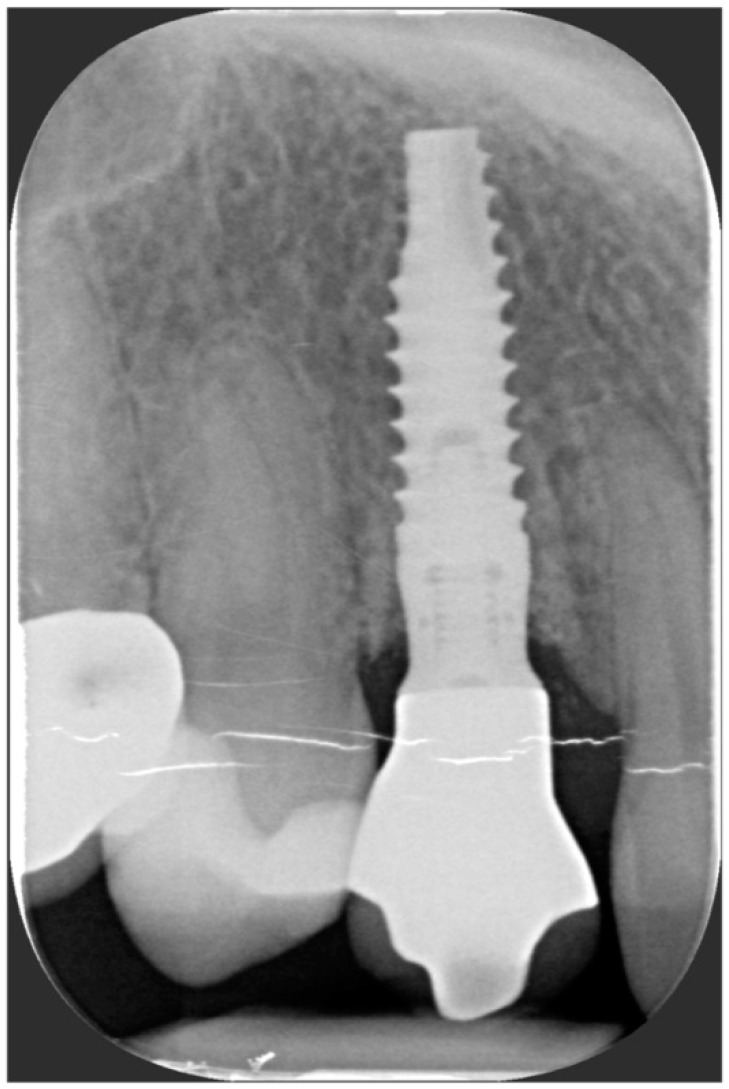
Marginal bone loss evaluated with periodical X-ray scans was minimal.
